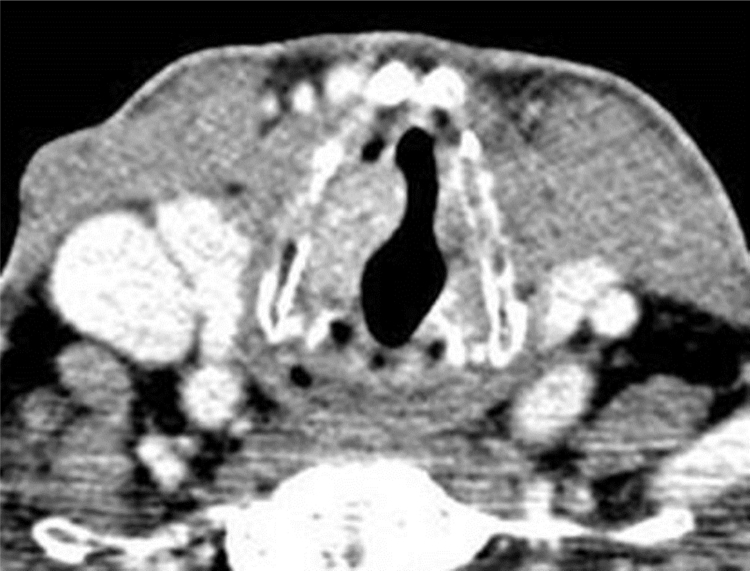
Contrast-enhanced computed tomography demonstrates an enhancing right laryngeal mass deeply located in the vocalis muscle.
